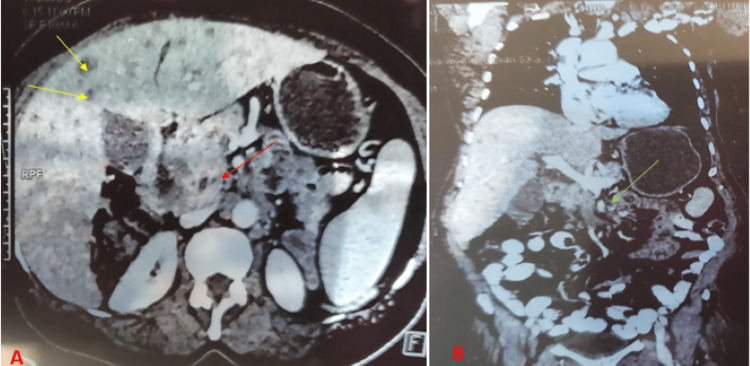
Computed tomography (CT) of the abdomen and pelvis A) Axial CT scan demonstrating duodenal mass with invasion of the pancreas (red arrow) and metastatic liver lesions (yellow arrow). B) Coronal CT image demonstrating thrombus of the superior mesenteric vein (green arrow).
